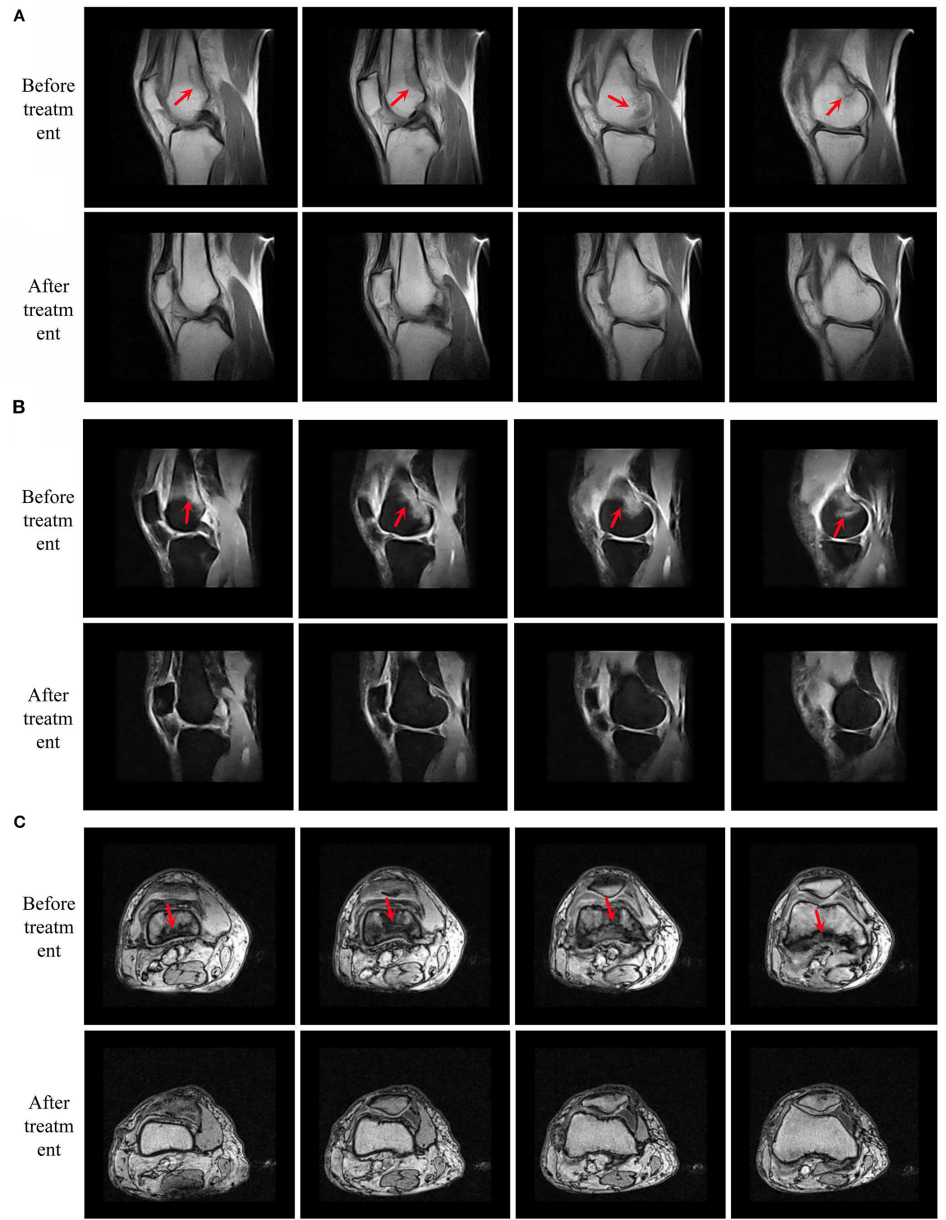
MRIs compared before and after treatment. Present four consecutive magnetic resonance images from left to right. (A) Sagittal conventional sequence. (B) Sagittal fat-suppressed sequence and (C) axial sequence. Serious bone marrow edema-like lesions (the red arrow). MRI, magnetic resonance imaging.
